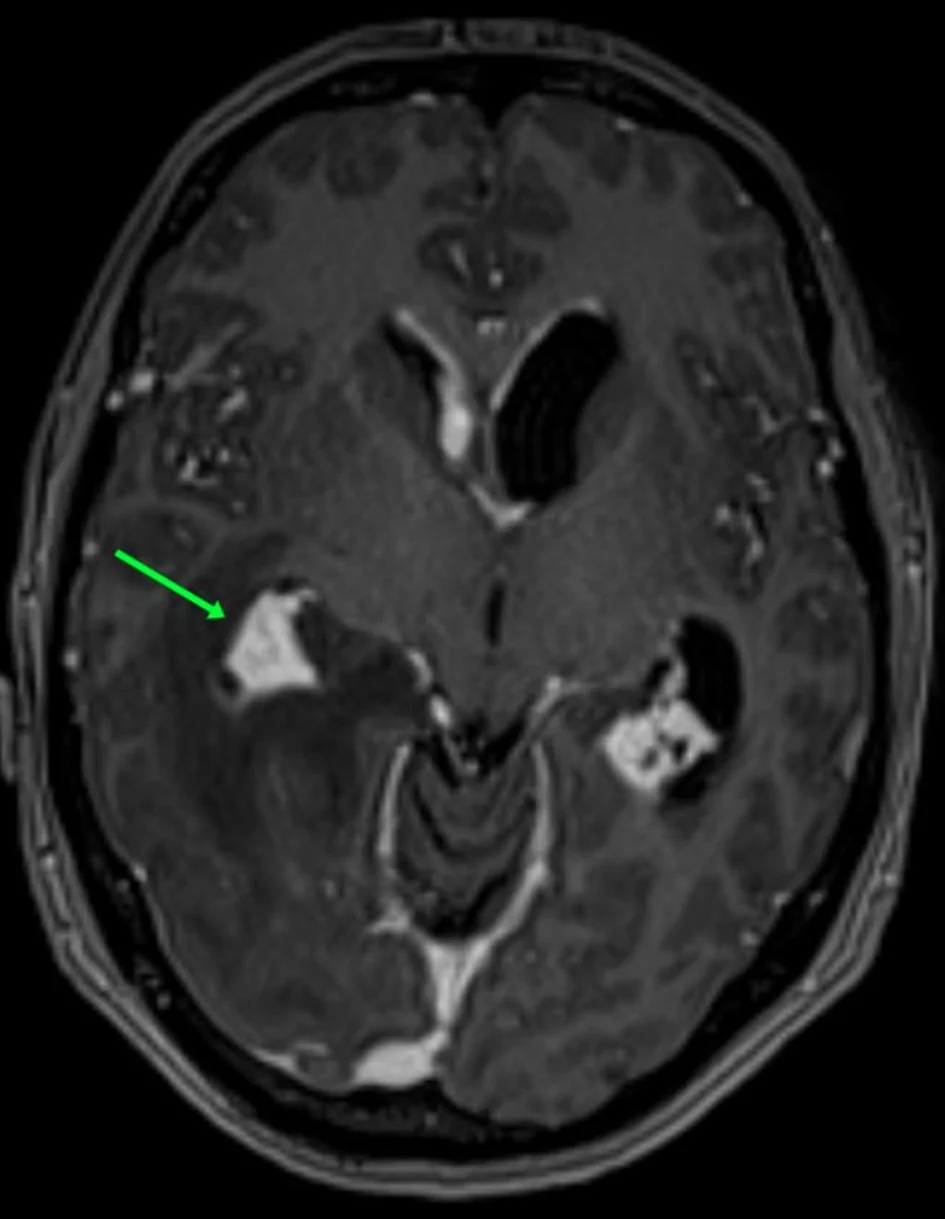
Post-contrast axial T1-weighted MRI demonstrating extension of the lesion into the posterior and temporal horns of the right lateral ventricle, with near-complete obliteration and distortion of the ventricular lumen (green arrows). MRI: magnetic resonance imaging.
